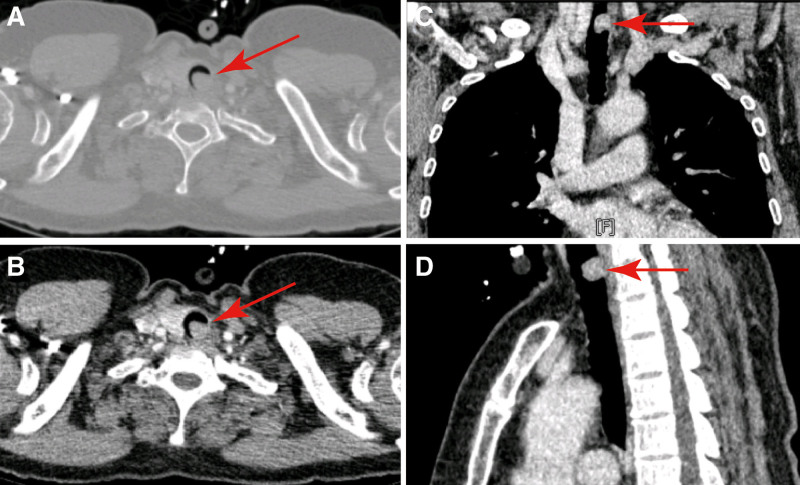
CT scan shown a 1.34 × 1.23 cm sized protruding mass in the left posterior wall of the superior trachea resulting in a 90% obstruction of trachea lumen. (A) Transverse CT lung window setting images; (B) post-contrast enhanced image; (C) coronal post-contrast enhanced image; and (D) sagittal post-contrast enhanced image.
